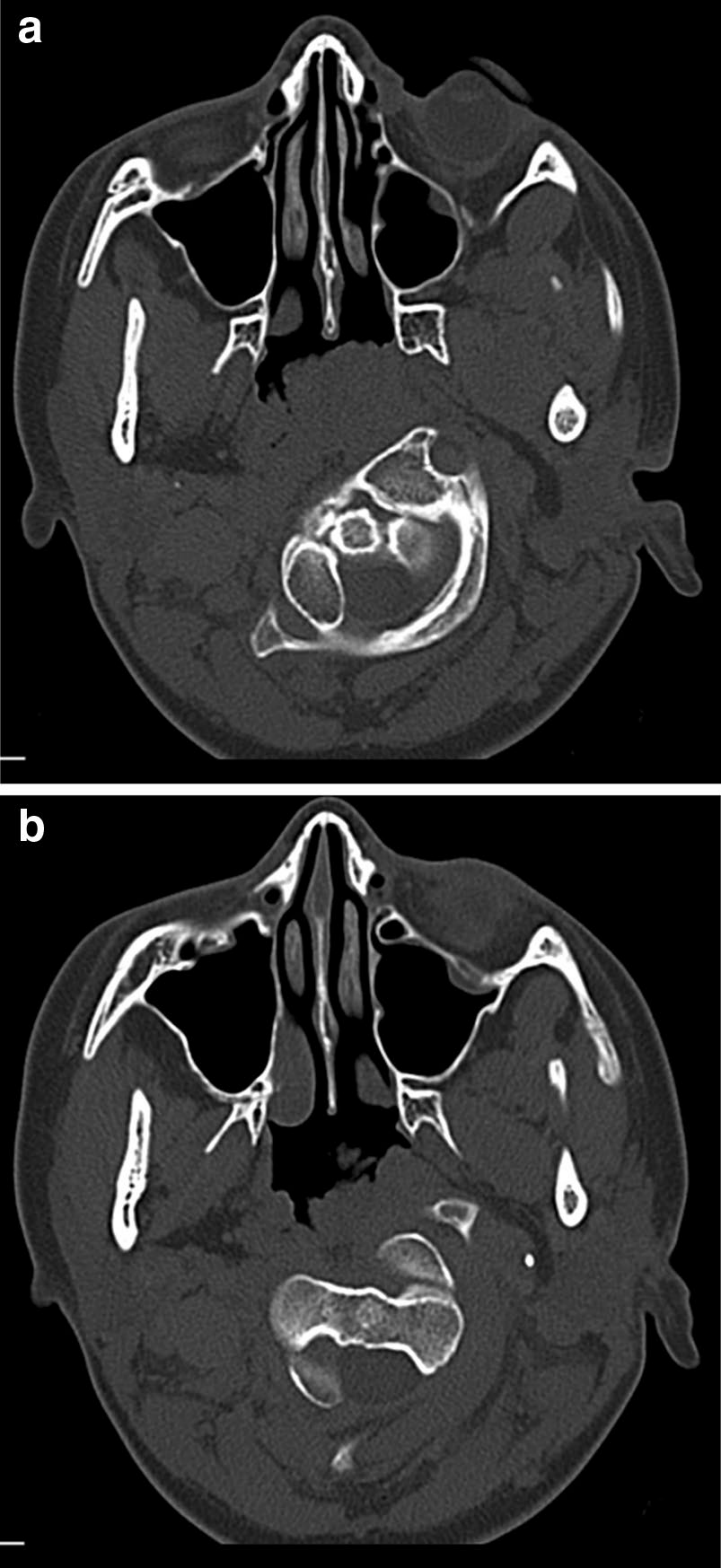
a, b Axial CT images demonstrating rotatory subluxation of C1 on C2. The left lateral mass is located anterior and lateral to its normal position and the right lateral mass is located posteriorly and medially
